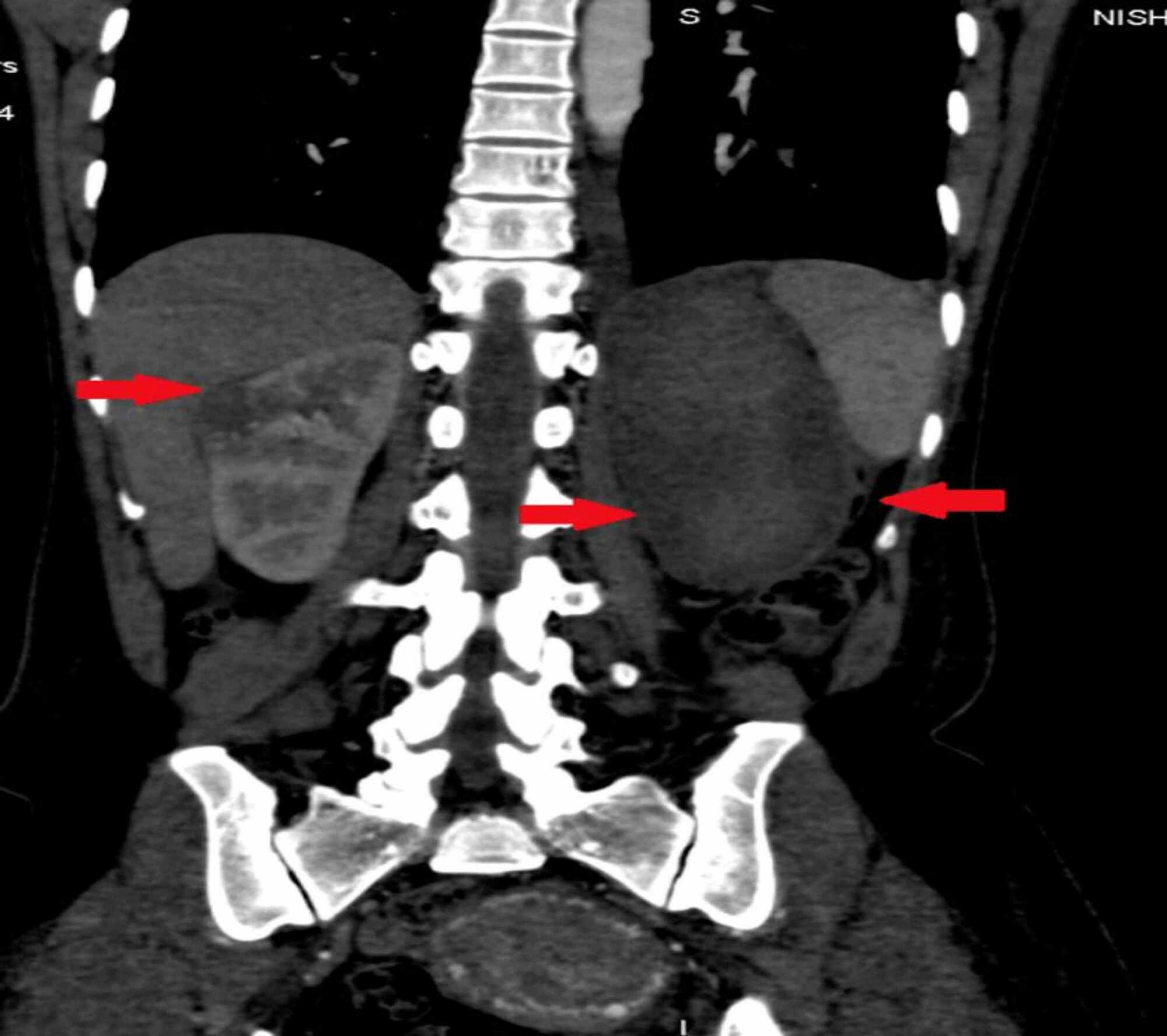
CT abdomen coronal reformat showing a soft tissue density mass in the kidney and large subcapsular collection (hematoma) in the left kidney CT: computed tomography
